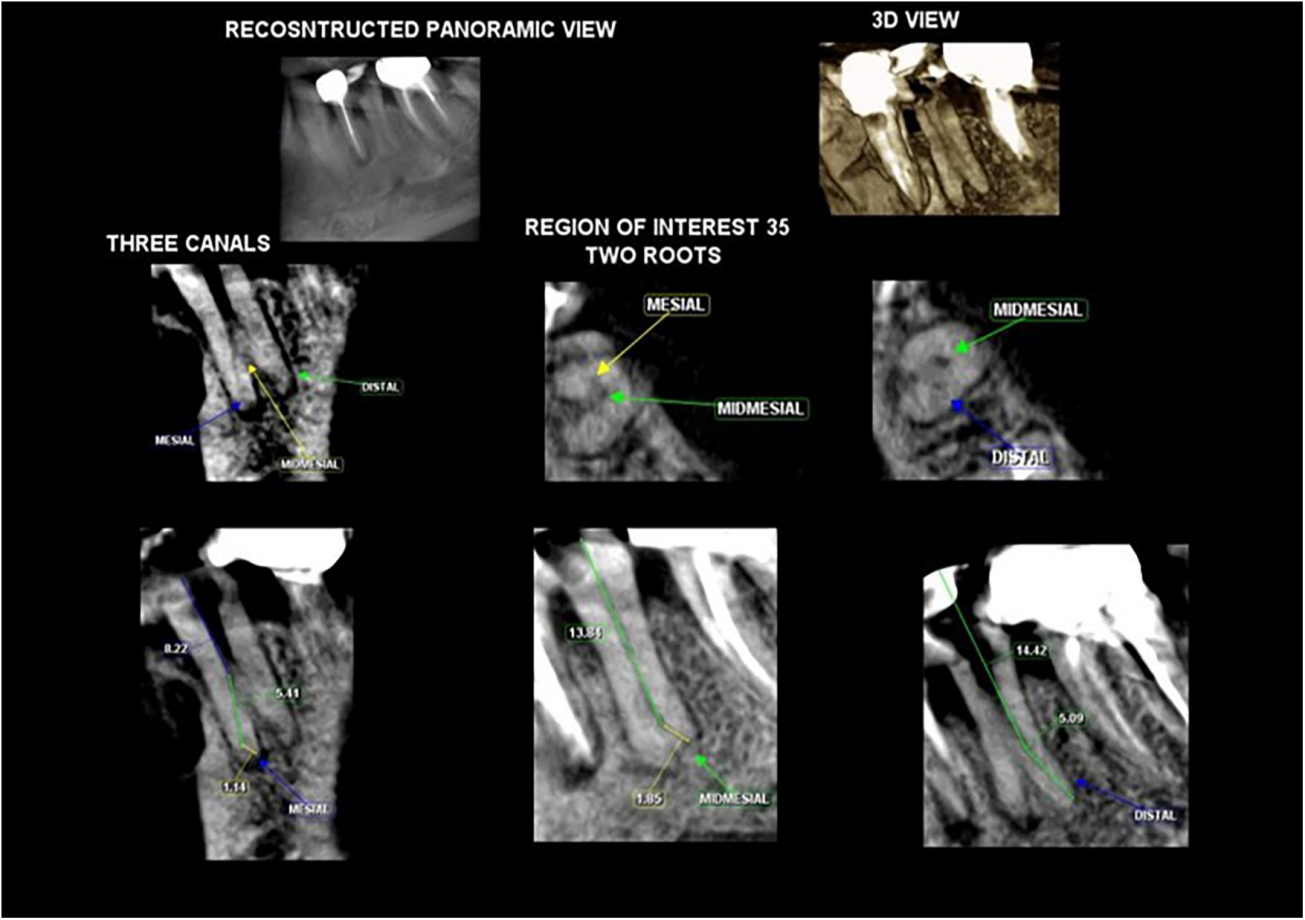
CBCT confirming the presence of 2 roots and 3 canals.
